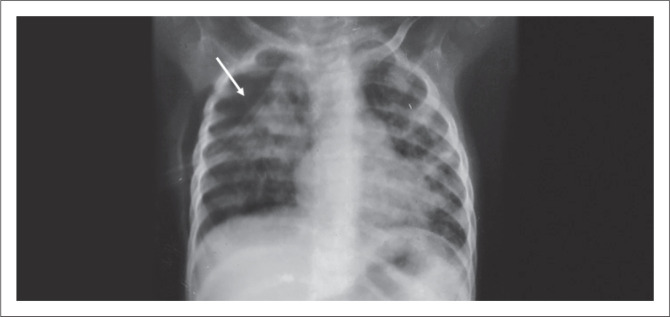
An 18-month-old male, follow-up case of disseminated multidrug resistant (MDR) TB on MDR regime. Chest radiography shows multiple cystic lucencies, ill-defined opacities in both lungs and a right pneumothorax with an intercostal drainage tube in situ (arrow).
